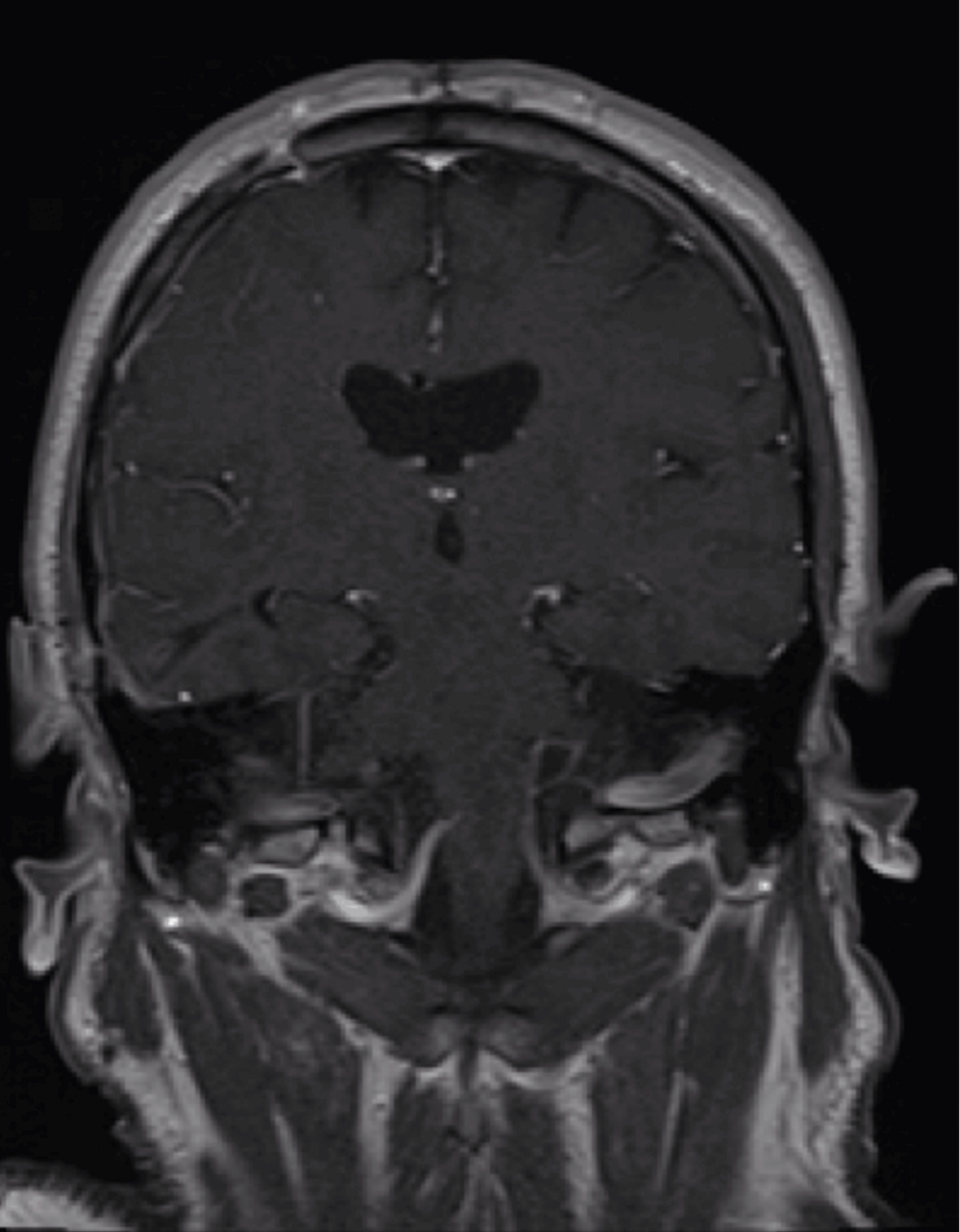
Six-month Interval MRI Brain MRI brain six months after clip ligation of CSF-venous fistulas reveals persistent resolution of subdural hematoma and leptomeningeal enhancement MRI - Magnetic Resonance Imaging CSF - Cerebrospinal Fluid
